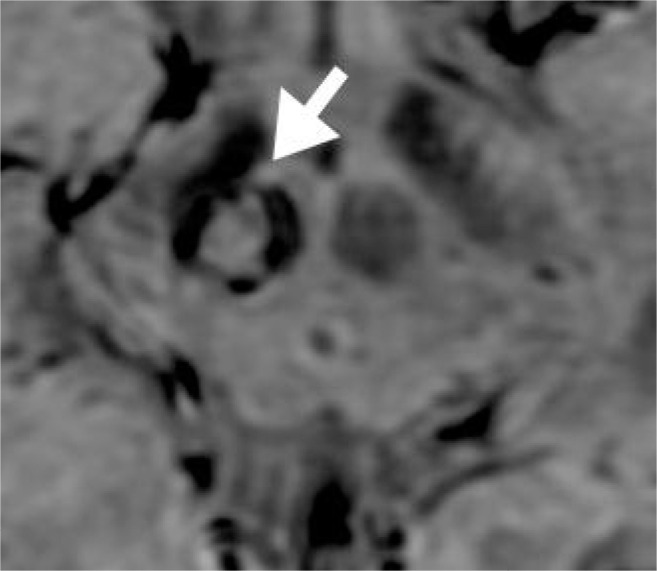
MRI scan of a patient with cerebral toxoplasmosis. Axial SWI showing
